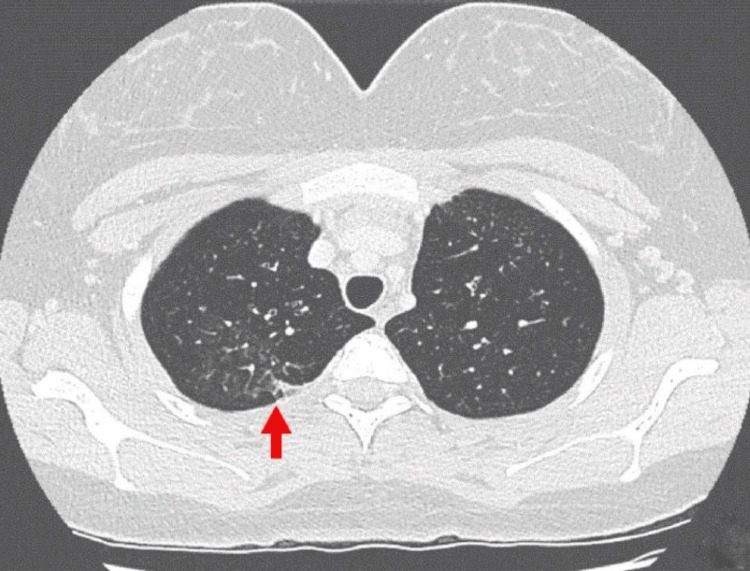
Chest computed tomography of Case 1 showing benign-looking subpleural nodules (arrow).
